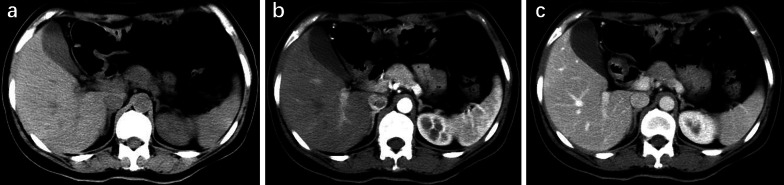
MiNEN of the pancreatic neck in a 48-year-old woman. a Unenhanced CT scan shows an ill-defined mass. The CT value of the tumor is 42 HU. b Arterial phase CT scan shows diffusely moderate homogeneous enhancement of the purely solid lesion with a size of 2.5 cm. The CT value of the tumor is 81 HU. c Portal phase CT scan shows washout of the oval tumor. The CT value of the tumor is 66 HU
